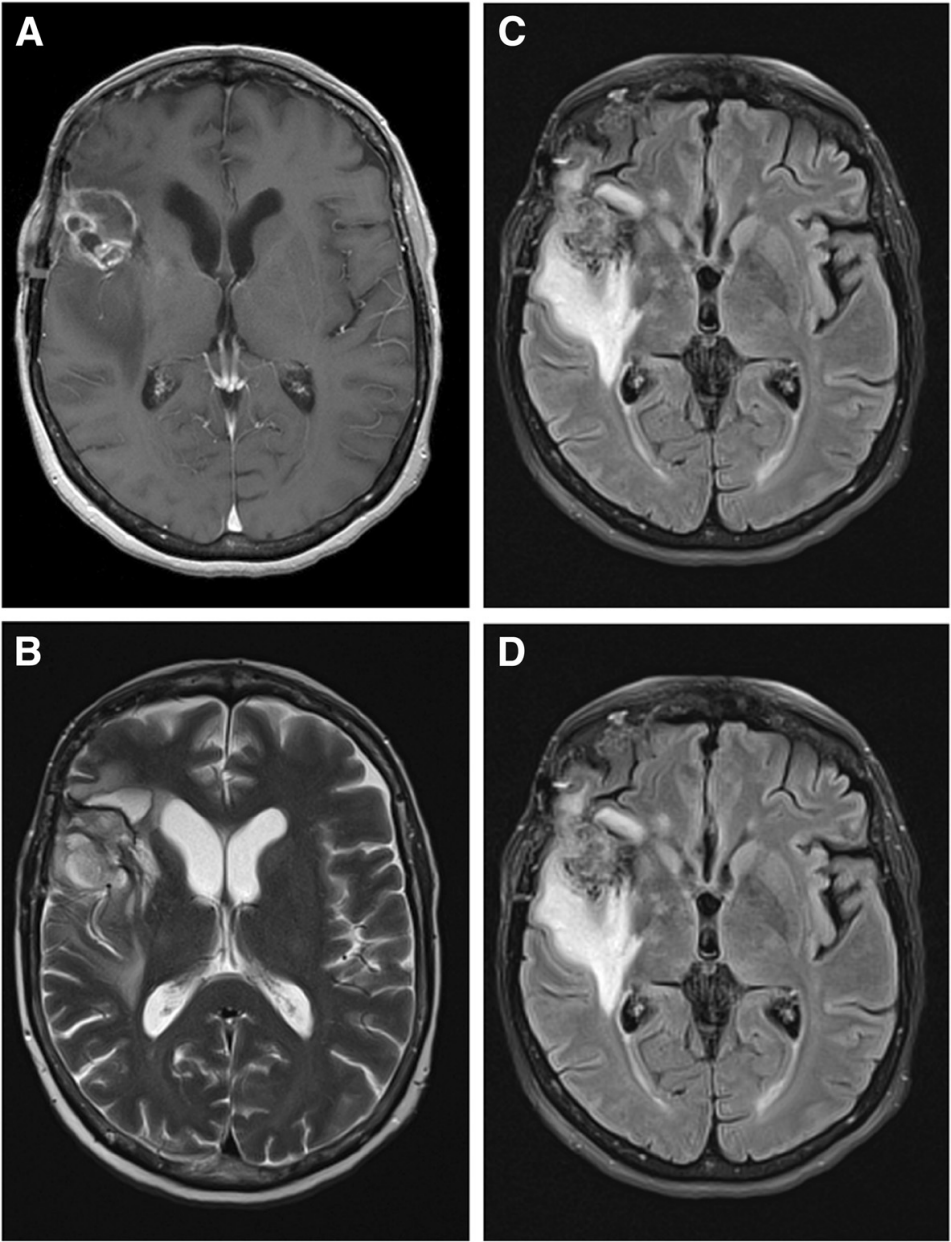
Radiological evaluation of the brain. T1-weighted axial gadolinium-enhanced magnetic resonance image demonstrates an enhancing tumour of the right frontal lobe (A). T2-weighted image demonstrates the same lesion as in the previous image, with notable tissue edema (B). This finding is consistent with a high-grade glioblastoma. On fast fluid-attenuated inversion-recovery (FLAIR) MRI scan a zone of edema is identified around the tumour demonstrating increased signal intensity (C, D).
